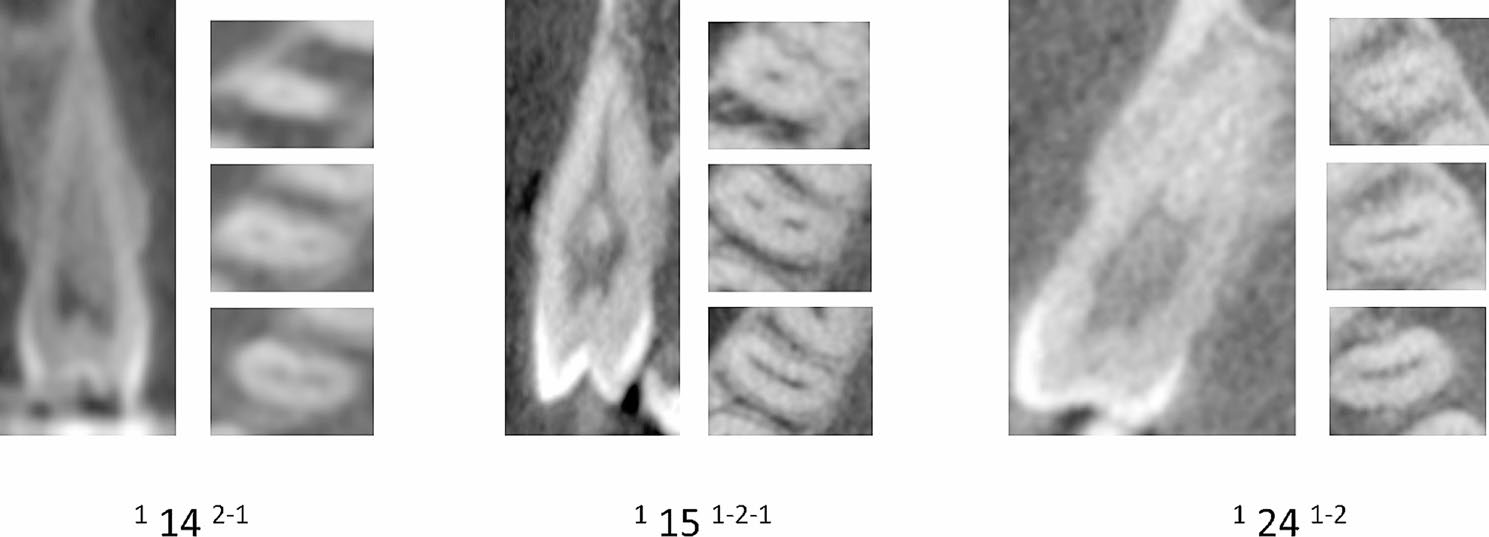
CBCT View (Sagittal and axial) maxillary first and second premolars showing the canal variations
